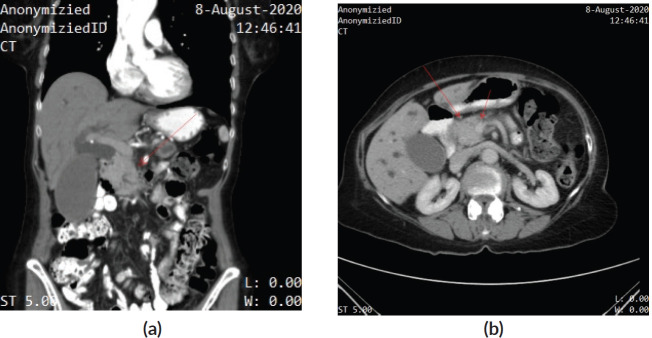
(a): Contrast-enhanced CT – coronal section – showing a mass at the head of the pancreas, encasing the superior mesenteric vein. (b): Contrast-enhanced CT showing a mass at the head of the pancreas, encasing the superior mesenteric vein.
